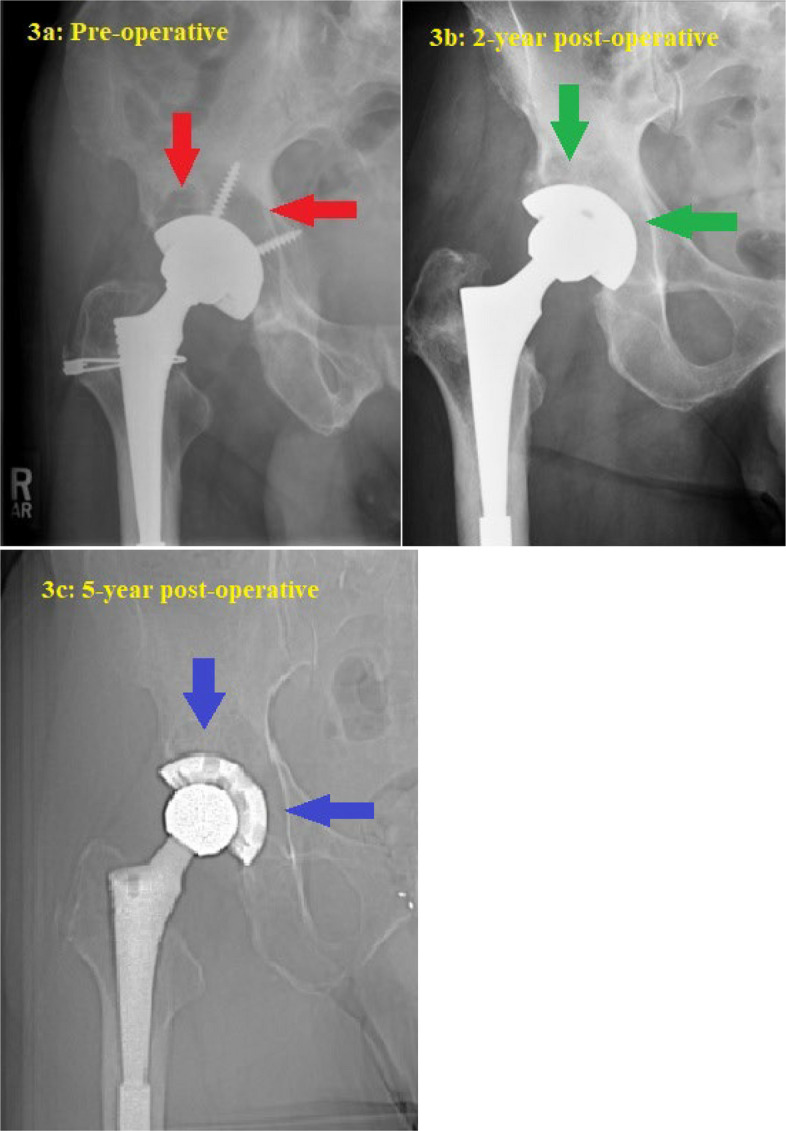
Serial imaging of a 74-year-old male following isolated liner and head exchange for retro-acetabular osteolysis, with retention of the well-fixed cup and stem. All screws were removed for stability testing and to access the retro-acetabular region for grafting with demineralized bone matrix using a vinyl urinary catheter and syringe. a Pre-operative radiograph showing retro-acetabular osteolysis (red arrows); b Two-year post-operative radiograph demonstrating the incorporation of demineralized bone matrix (green arrows) in the previous osteolytic lesion; c Five-year post-operative CT scout view revealing near-complete resolution of the retro-acetabular osteolysis (blue arrows)
